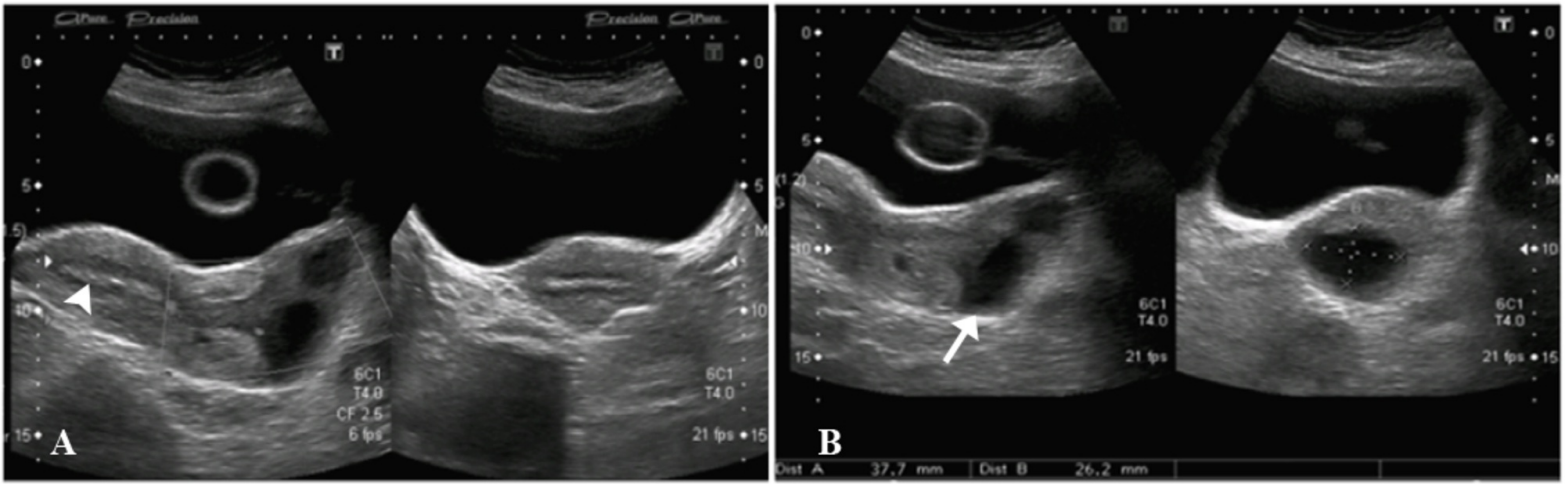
Transabdominal ultrasound images. A) Uterine fundal endometrium appears normal (arrowhead). B) Heterogenous area (arrow) in the superior one-third of vagina with two anechoic cystic spaces within it containing internal echoes. Foley's catheter balloon noted within the urinary bladder.
